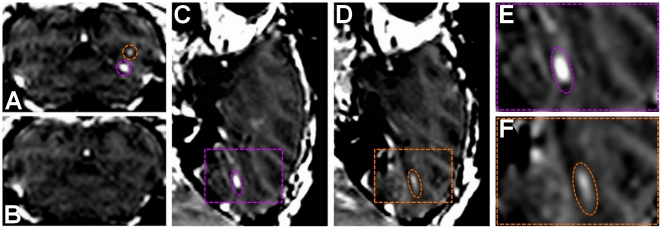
BBB opening in V3. A 3D Spoiled Gradient-Echo (SPGR) T1-weighted sequence was applied after intravenous (IV) injection of gadodiamide 1 h after sonication. Gadodiamide presence in the brain parenchyma is induced by BBB opening. (A) Coronal MR images confirming the local disruption of the BBB, two opening sites are circled confirmed by the diffusion of the contrast agent (purple circle 0.3 MPa and orange circle 0.45 MPa). (B) Coronal MR images 6 days, after sonication, confirming closing of the BBB and proving reversibility of the procedure. (C,D) Sagittal MR images of the two sonication sites at two different pressures 0.3 MPa (C) and 0.45 MPa (D). (E,F) Magnification of the corresponding color boxes.
